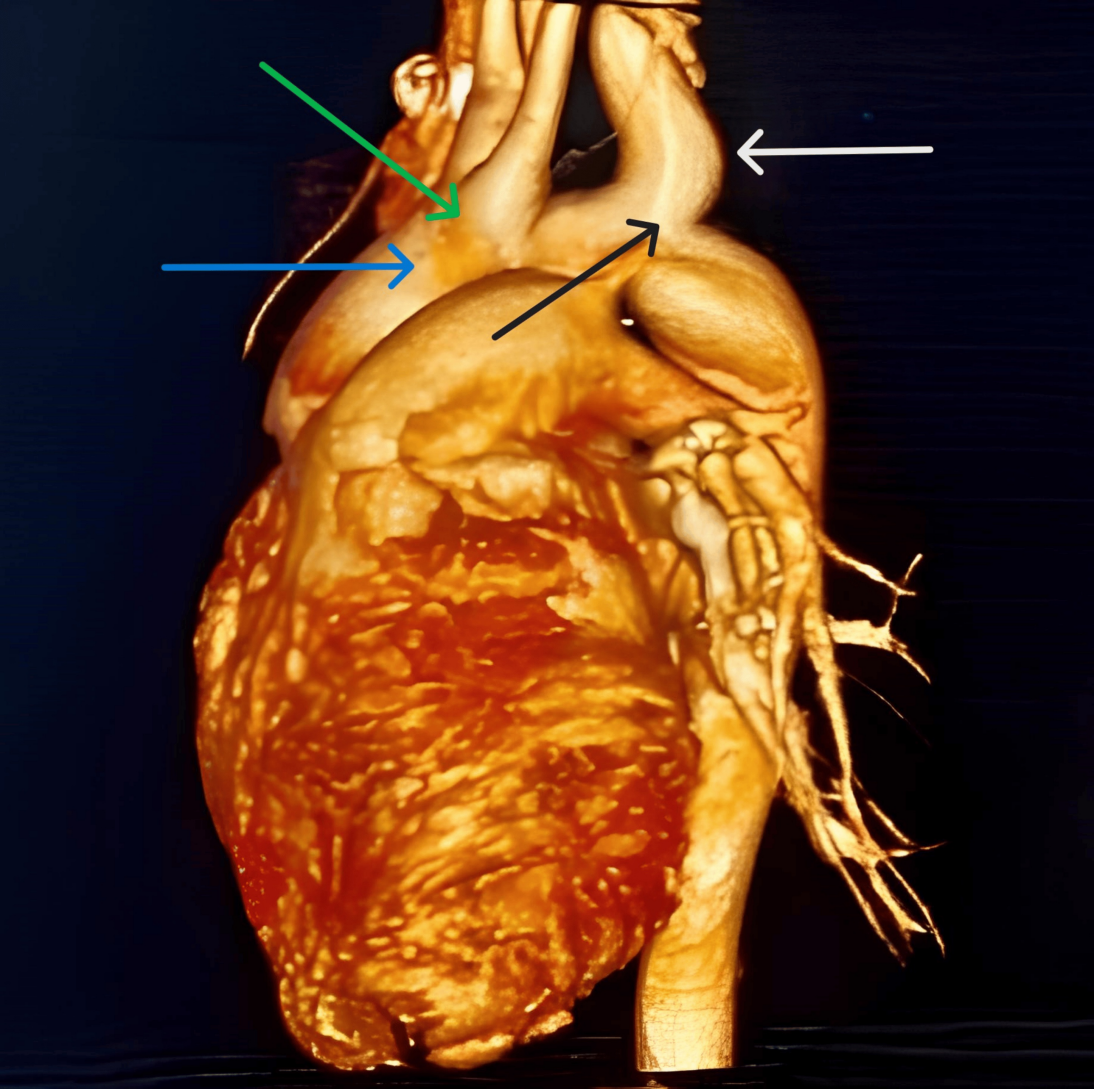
Computed tomography (CT) aortogram showing arch of aorta (blue arrow), common origin of right brachiocephalic and left common carotid artery (green arrow), aneurysmal dilatation of left subclavian artery (white arrow), coarctation of aorta (black arrow).
